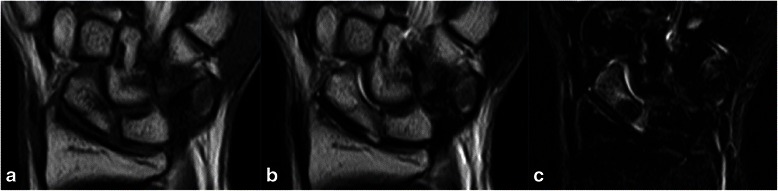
Magnetic resonance imaging scans obtained 2 weeks after the patient’s initial presentation. Low intensity can be seen on the T1-weighted image (a), isointensity to high intensity on the T2-weighted image (b), and low intensity on the T2-weighted image, with fat suppression (c) at the waist of the scaphoid
